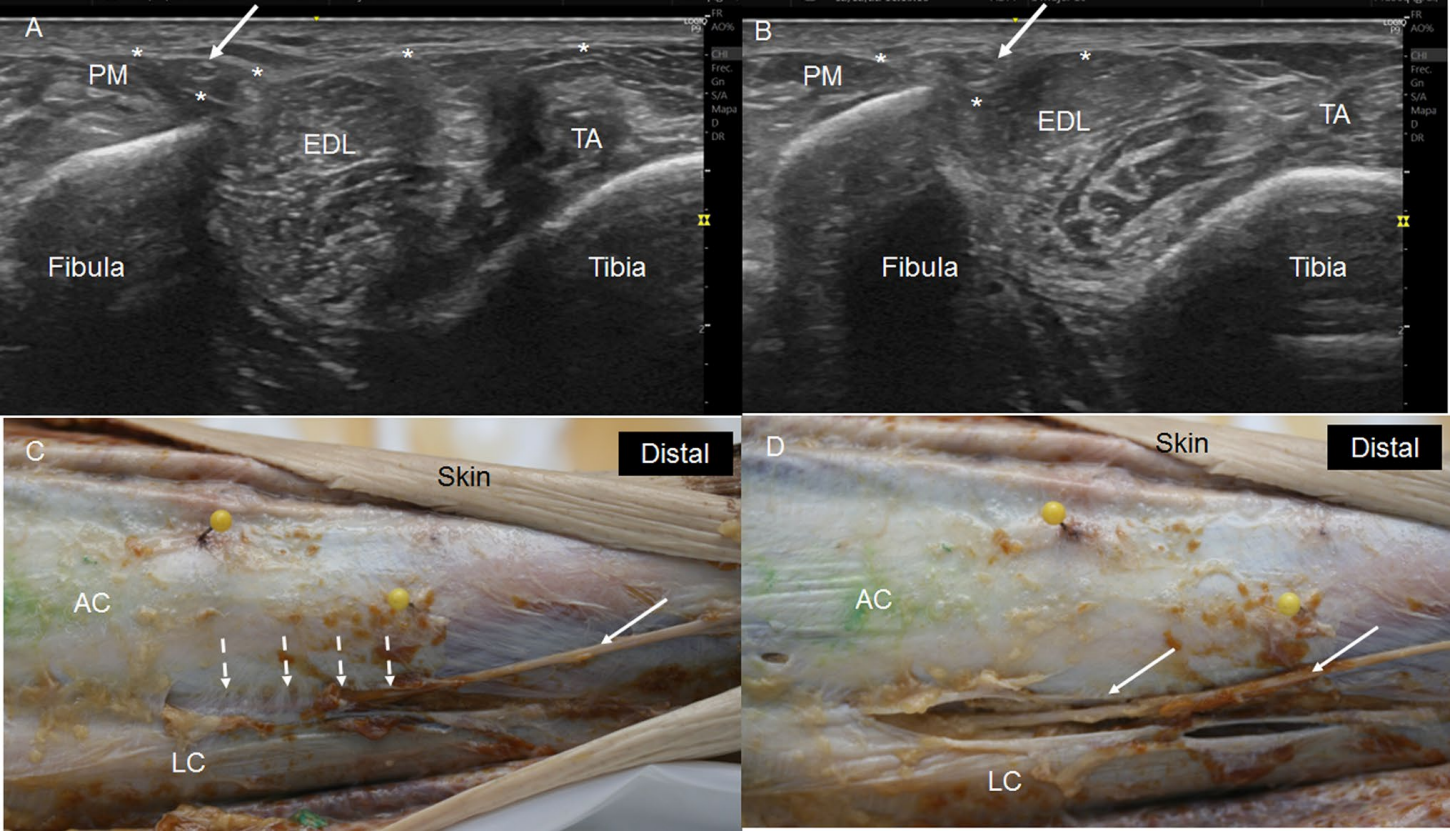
The exit of the superficial fibular nerve (SFN). A Ultrasound view of the SFN (white arrow) inside the tunnel that crural fascia (white asterisk) makes for the nerve, medially to the peroneus muscles (PM). Extensor digitorum longus (EDL) and tibialis anterior (TA) muscles covered by the crural fascia (white asterisks) B Ultrasound view of the exit of SFN (white arrow) after crossing the crural fascia (white asterisk). C Dissection of the leg shows the crural fascia under the skin forming the anterior (AC) and the lateral (LC) compartment of the leg. Yellow needles, medially located, and placed thanks to the previous ultrasound study, mark the tunnel under the crural fascia for the SFN (white intermittent arrows) D Exposition of the SFN inside the tunnel (white arrows), after cutting the crural fascia
